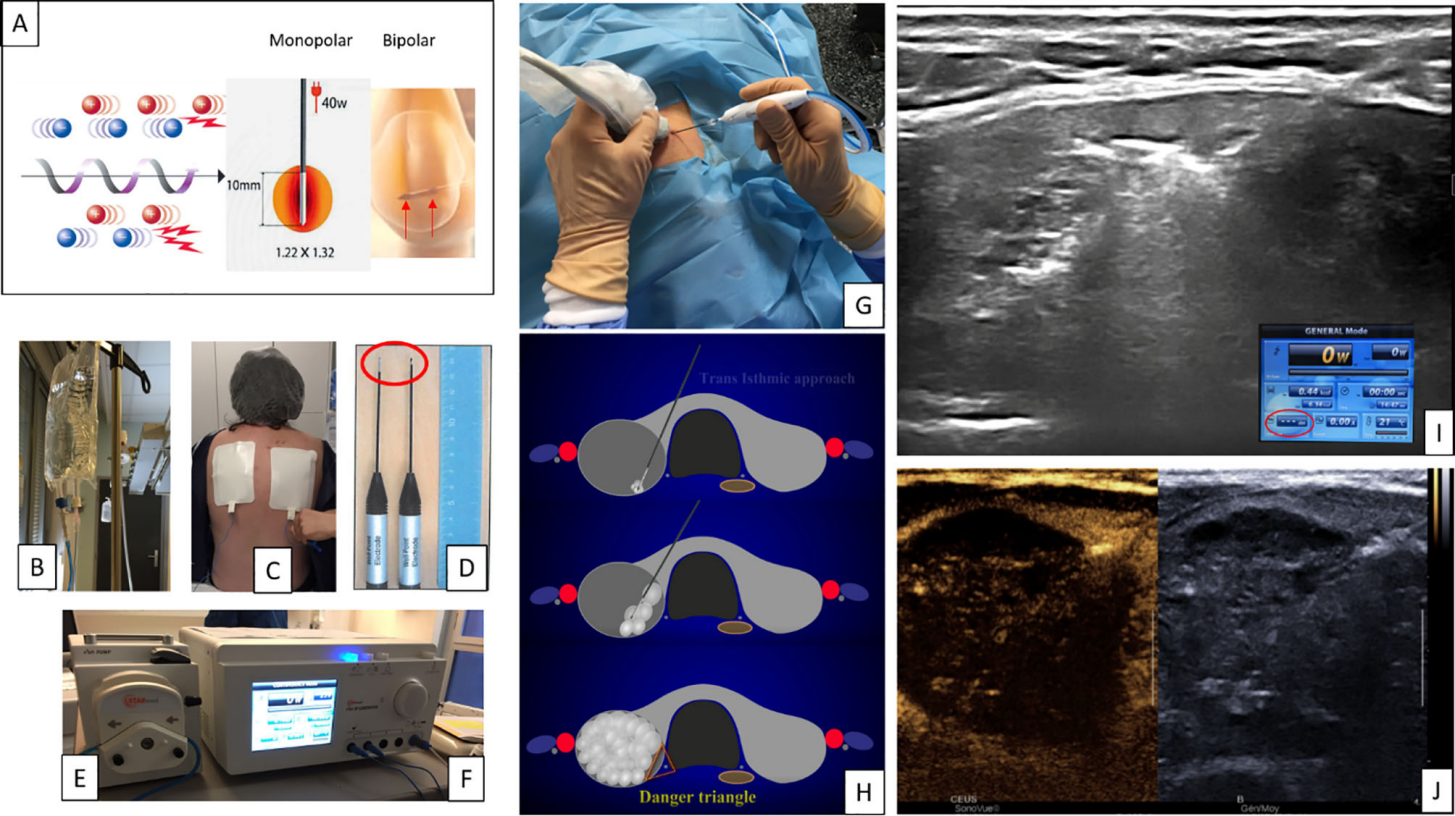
Radiofrequency ablation (RFA): physiology, equipment and ablation. Ionic agitation (A) and formation of frictional heat. Monopolar: Using a 40W RF power, and an active-tip of 10 mm, the diameter of the action zone is 13 mm. Bipolar RFA electrode with two tips (D). Internally cooled electrode (active-tip 5 and 10 mm) receives cold fluid (B) perfusing by a peristaltic pump (E). The generator (F) supplies RF power through the active-tip of the electrode. Grounding pads (C) act to disperse electricity in the RF circuit. Using ultrasound guidance (G), the electrode is inserted into the nodule. A trans-isthmic approach (H) and a moving shot technique are recommended. A cloudy effect (I) and an increase of the impedance (red circle) indicate the moment to change ablation zone. CEUS confirms (J) the complete treatment of the nodule (“vascular desert”).
